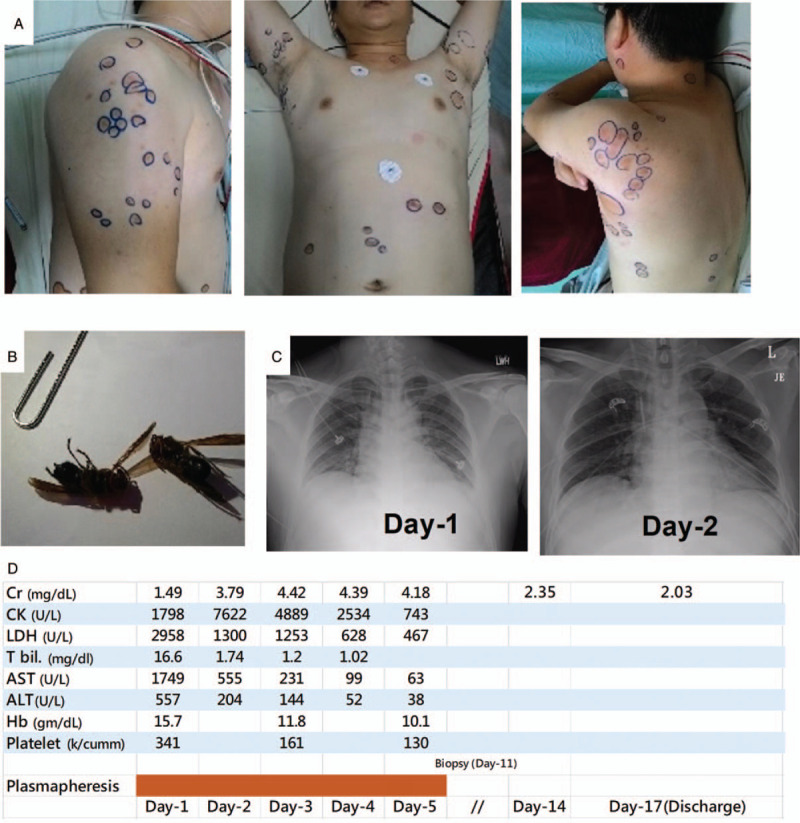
Skin lesions on the trunk (A), 2 captured dead wasps (B), serial chest X-ray (C), and serial laboratory findings and management (D) of case 1. ALT = alanine aminotransferase, AST = aspartate aminotransferase, CK = creatine kinase, Cr = creatinine, Hb = hemoglobin, LDH = lactate dehydrogenase, Plt = platelet.
